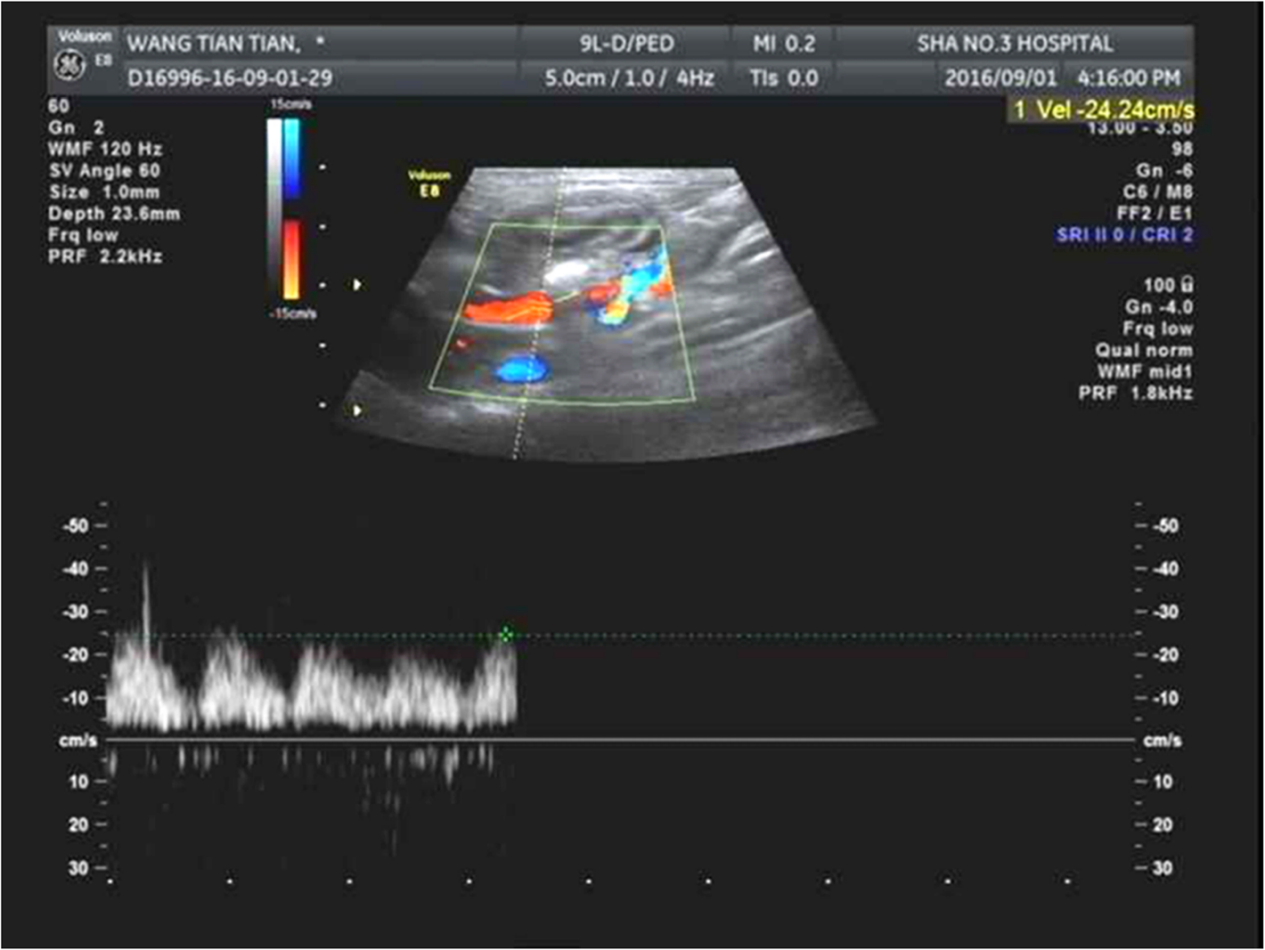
The Doppler ultrasound image of the portal venous system
